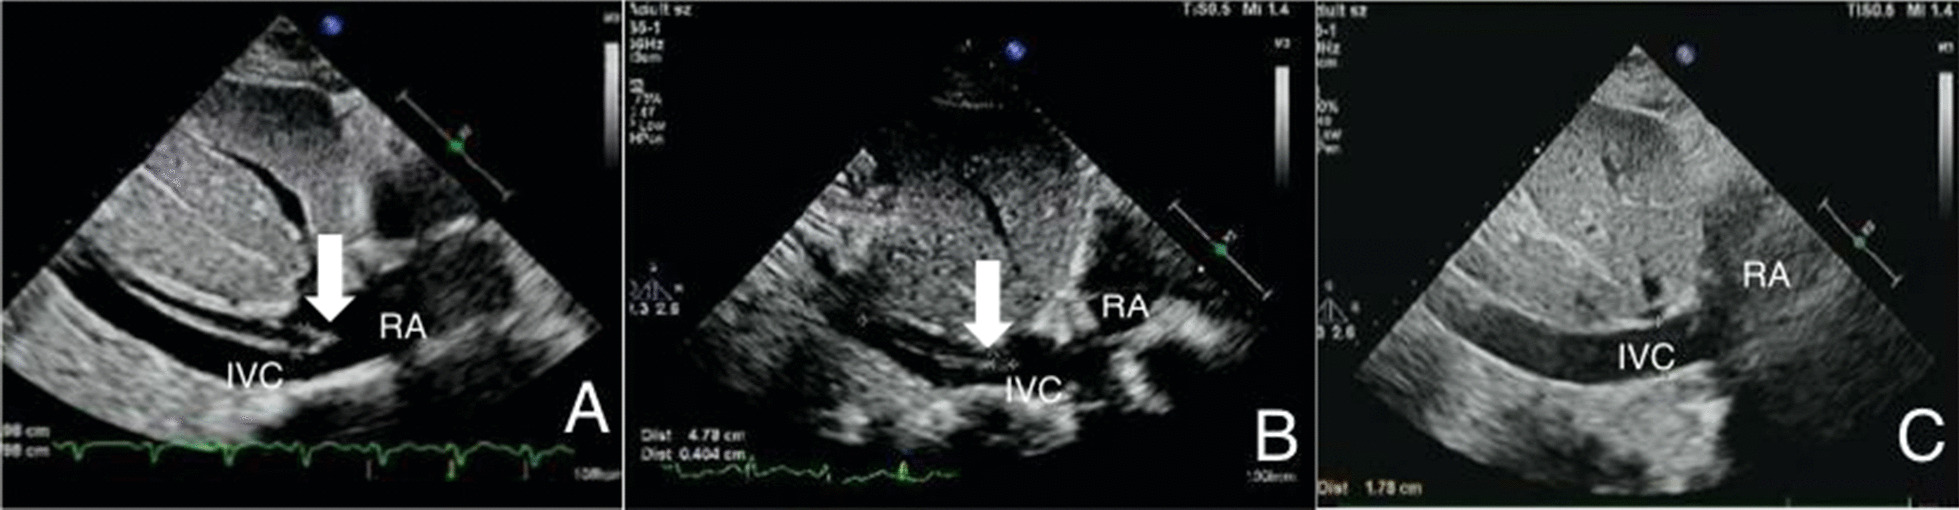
Echocardiography after cannula removal. a shows that after extracorporeal membrane oxygenation catheter removal, the inferior vena cava thrombosis was found by ultrasonography. b shows that the thrombosis of the inferior vena cava decreases gradually after heparin anticoagulation. c shows that the thrombus of inferior vena cava disappeared after heparin anticoagulation. *RA* right atrium, *IVC* inferior vena cava, Thrombosis in   inferior vena cava (arrowhead)
